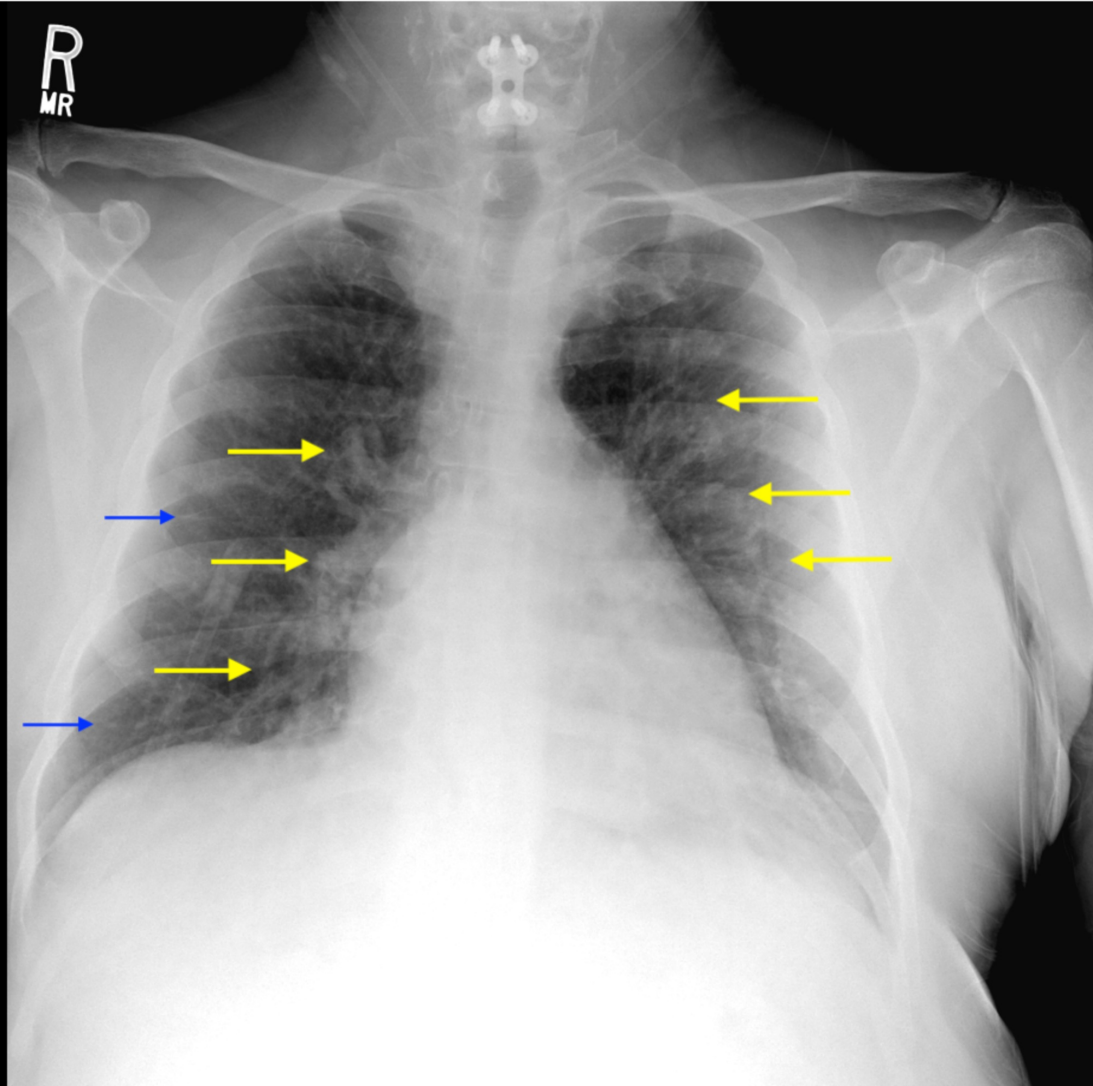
Chest X-ray of the patient The image displays the development of diffuse pulmonary interstitial edema/infiltrates, including perihilar vascular congestion (yellow arrows) and Kerley B lines (blue arrows)
